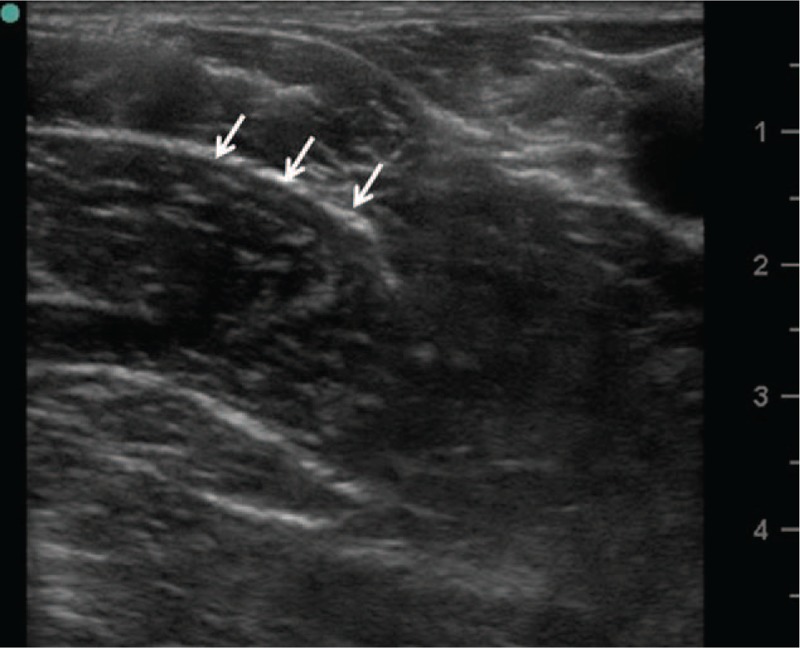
Ultrasound image of the anterior branch of the obturator nerve (arrows).
